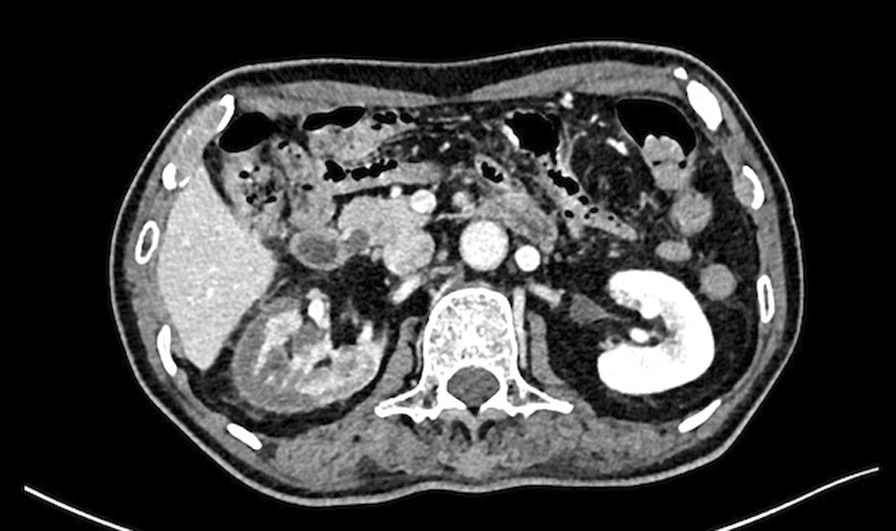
CT revealed multiple poorly-enhanced areas and a cortical rim sign in the right kidney
